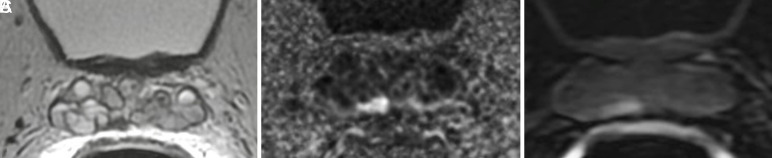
Magnetic resonance images of axial sequences T2WI (A), DWI (B), and Dynamic Contrast Enhanced (DCE) (C) of infiltration of seminal vesicles. Focal parietal thickening of a single seminal vesicle in the right, with low-signal intensity in the T2WI sequences; images of diffusion and perfusion, along with a hyperintensity in DWI and an early enhancement in the T1WI axial picture after contrast, support the result that we have shown in T2. DWI, diffusion-weighted imaging.
